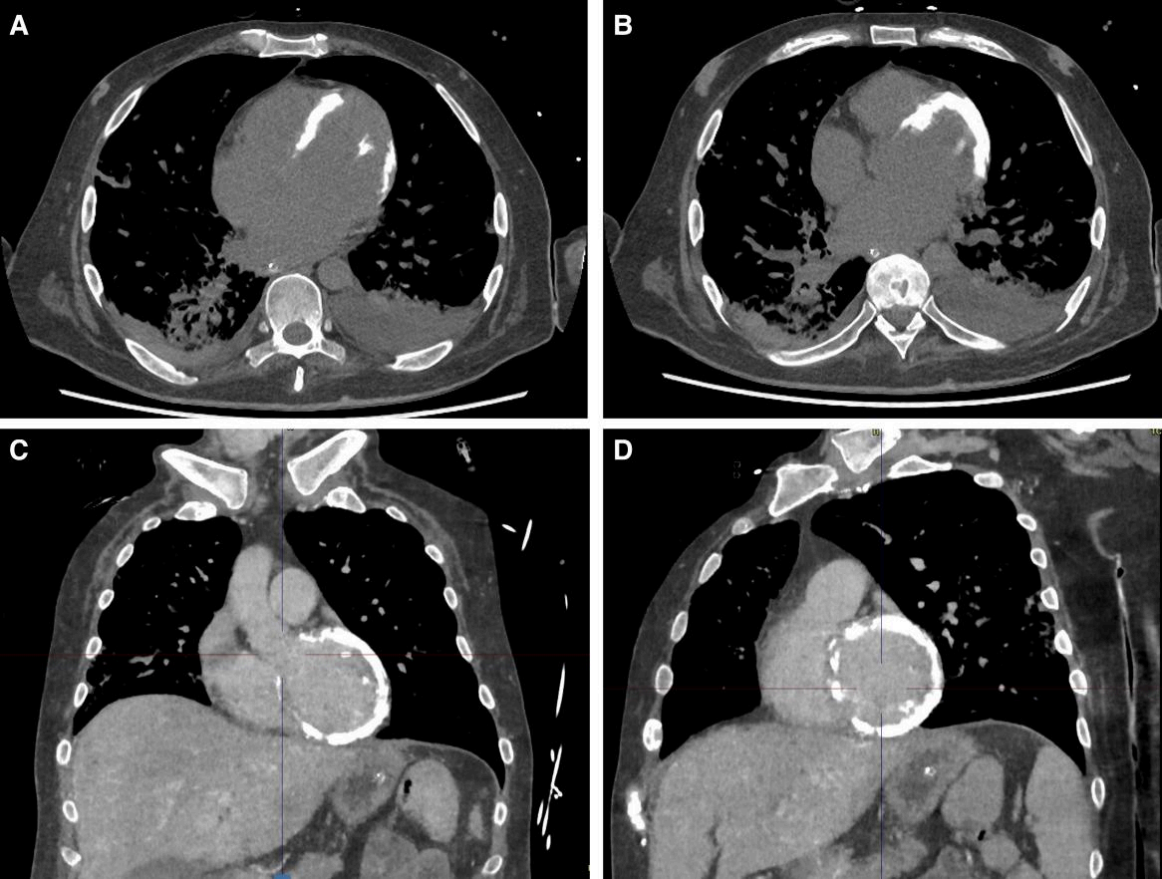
Non-contrast chest CT on day 70 of hospitalization showing extensive myocardial calcification. (*A–B*) Axial views and (*C–D*) coronal and sagittal reconstructions demonstrate diffuse epicardial and intramyocardial calcification involving the left ventricular wall. No calcification is observed in extracardiac structures. The maximum density measured was 359 Hounsfield units, consistent with calcium deposition.
